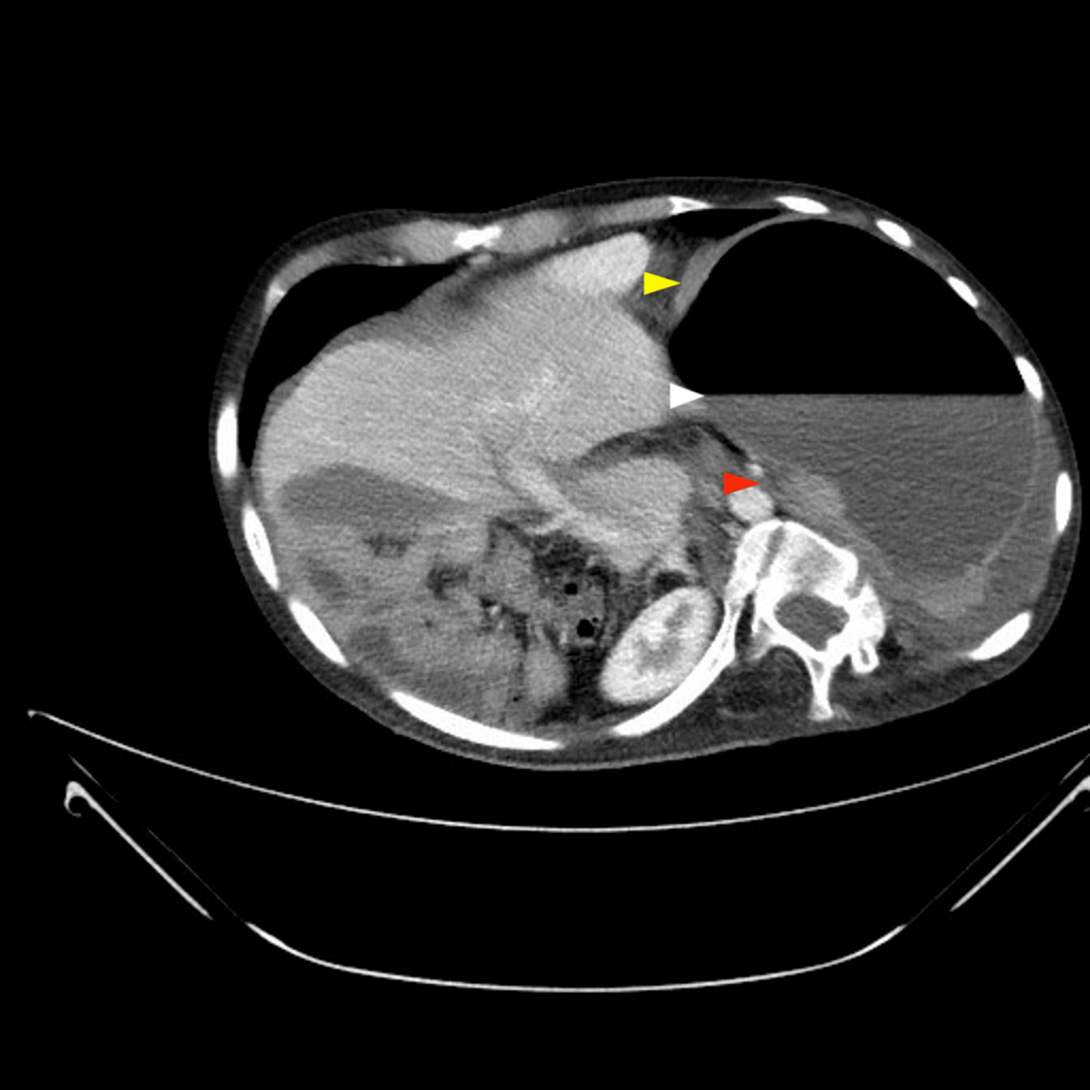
Computed tomography (CT) of the abdomen (axial view) Evident marked gastric dilation with a prominent fluid-air level (white arrow). The image shows the point of torsion (red arrow) of the gastric axis in the posterior-medial region, suggesting the whirlpool sign or a narrowing of the gastroesophageal junction. Additionally, gastric wall thickening (yellow arrow) is observed, consistent with congestion and potential ischemia.
